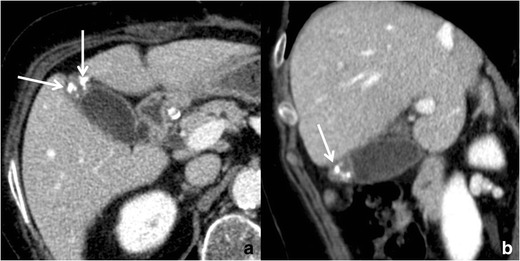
CT of gallbladder adenomyomatosis: intramural calcifications. CT accurately depicts intramural calcifications (*arrows*) that may develop within Rokitansky–Aschoff sinuses and which are pathognomonic for gallbladder adenomyomatosis
